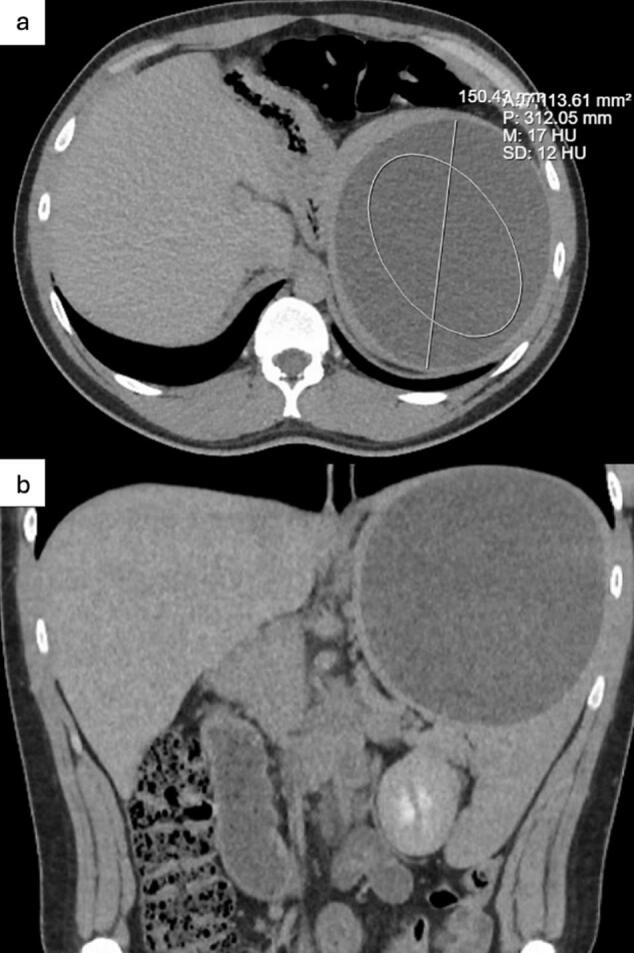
Contrast-enhanced Computed Tomography Angiography (CTA) of the abdomen incidentally revealing a large, hypoattenuating splenic lesion measuring approximately 15 cm in maximal diameter.
